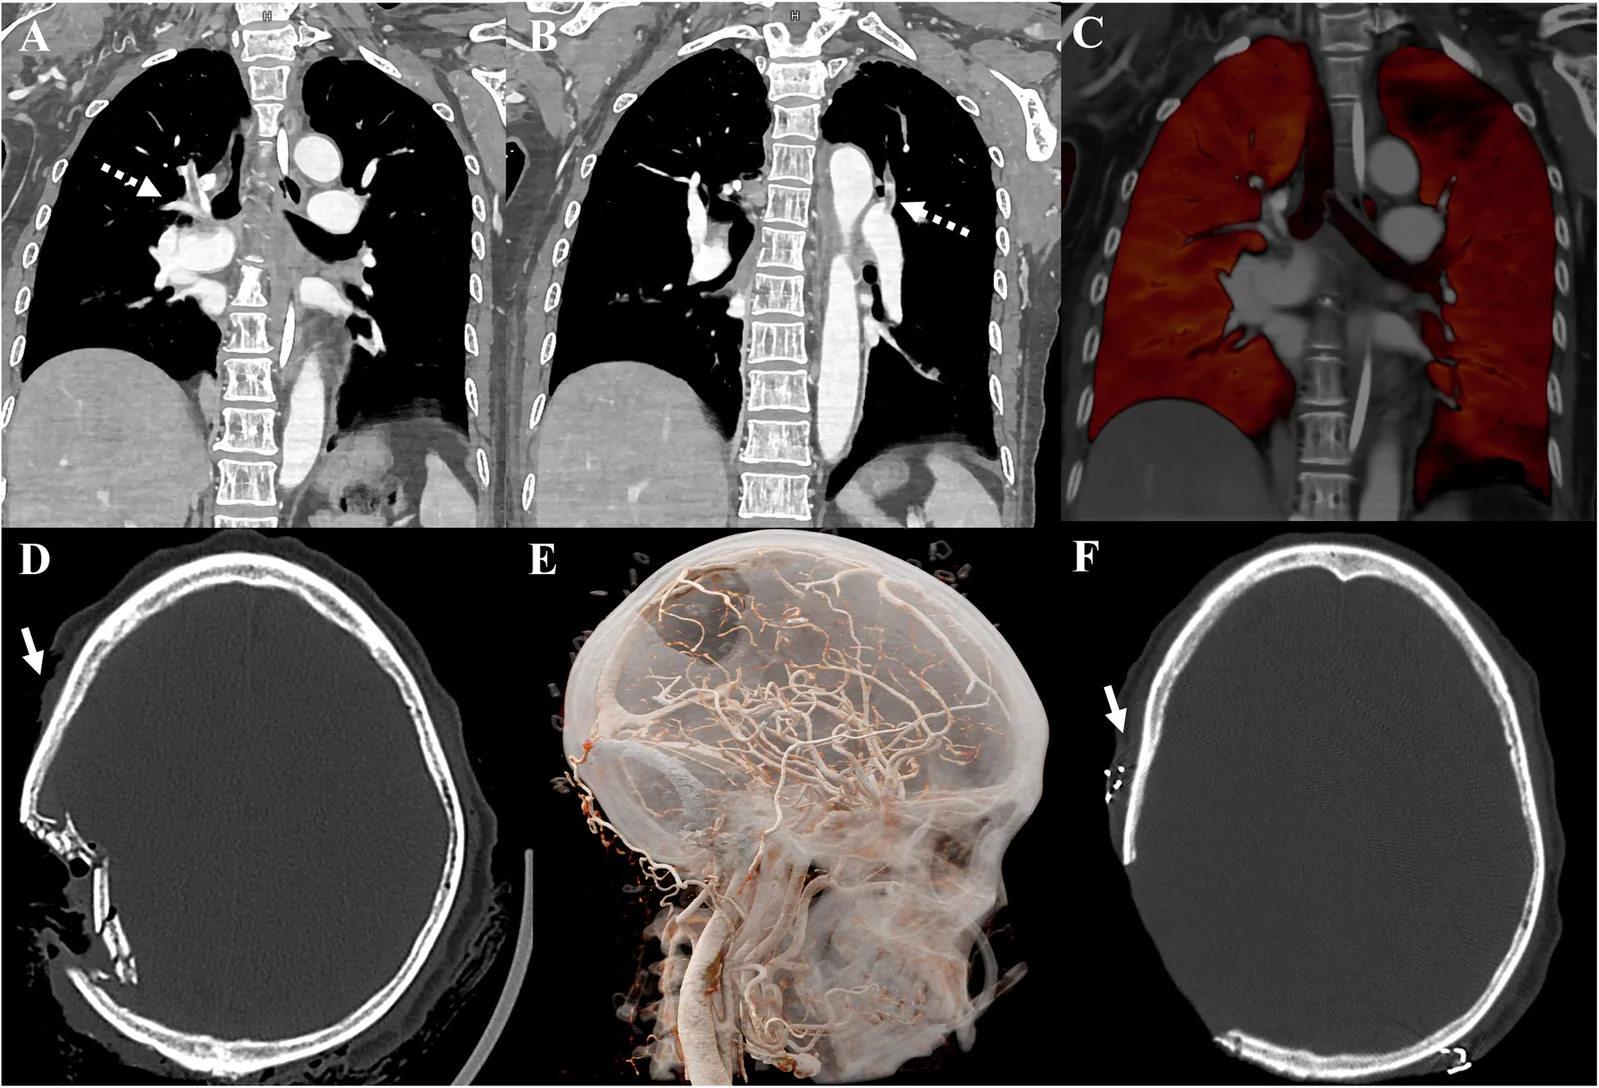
Pulmonary and cranial imaging of the trauma patient. (A,B) Coronal and axial CT pulmonary angiographic images demonstrate filling defects (white dashed arrows) in bilateral segmental and subsegmental pulmonary arteries, consistent with pulmonary embolism. (C) Iodine-density map in the coronal plane shows relatively preserved iodine distribution on the right lung (mean iodine concentration 1.82 mg/mL) and reduced iodine distribution on the left lung (mean 0.74 mg/mL); the color scale ranges from black (no iodine) to white (maximum iodine). Note that iodine density at a single arterial-phase acquisition is interpreted as a surrogate for pulmonary blood-volume distribution rather than as a direct measure of microvascular perfusion or gas exchange. (D) Axial image of the skull demonstrates the depressed right parietal fracture before surgery. (E) Cinematic volume-rendered image displays the course and distribution of the intracranial vessels after craniotomy for fracture elevation and epidural-hematoma evacuation. (F) Axial image of the head seven days after pedicled flap transplantation (white solid arrow) shows a viable, well-perfused flap.
